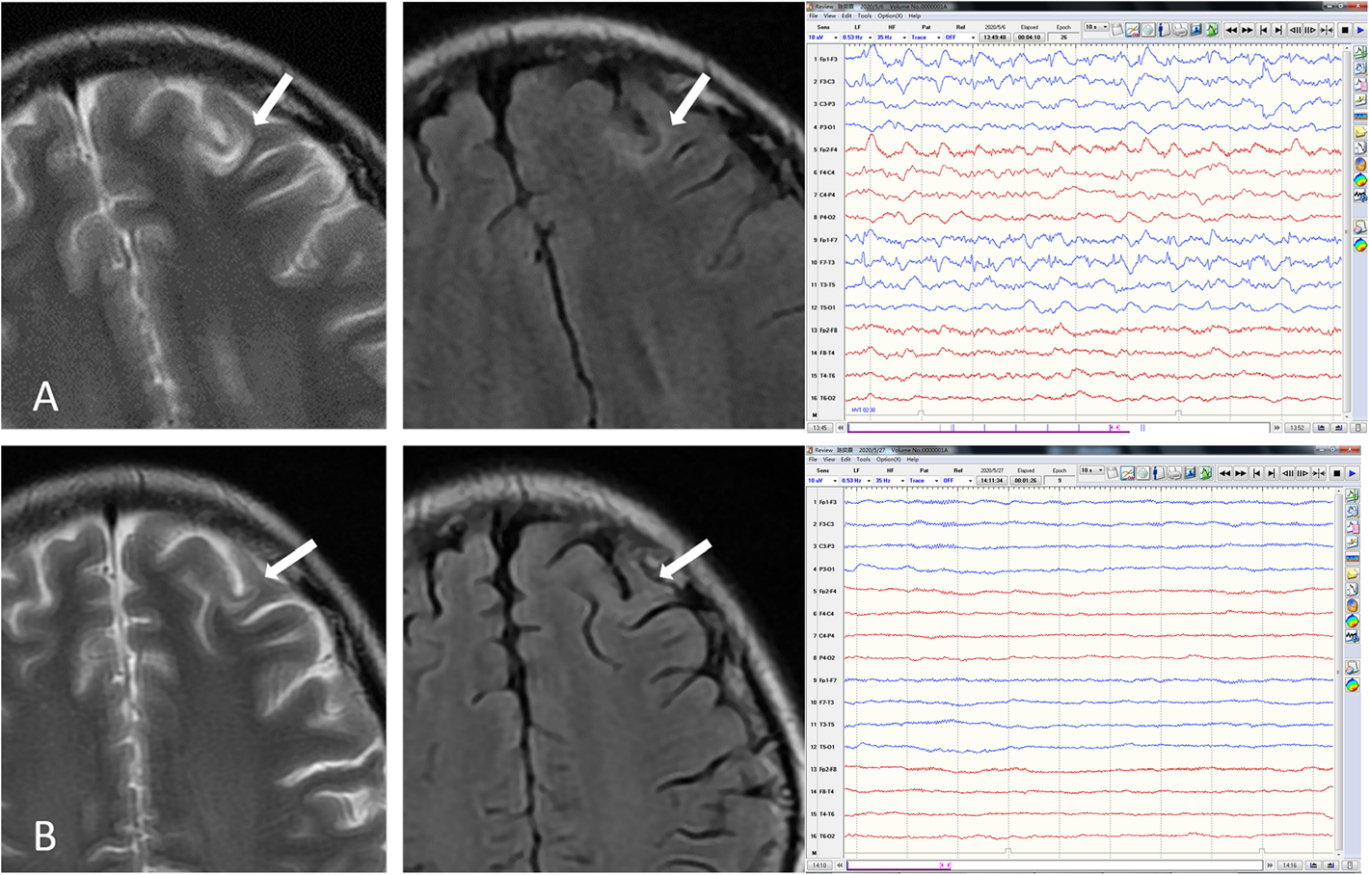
Brain MRI and EEG before and after treatment. Before treatment, T2WI and FLAIR (a, left and middle) showed a left frontal juxtacortical high signal intensity (upper arrows). EEG (a, right) revealed continuous 1.5 Hz spike wave complexes over the left frontal lobe. One month after admission, the lesion became faint on T2WI (b, left, arrow) and nearly undiscernible on FLAIR (B, middle, arrow). EEG (b, right) showed normal electrical activity
